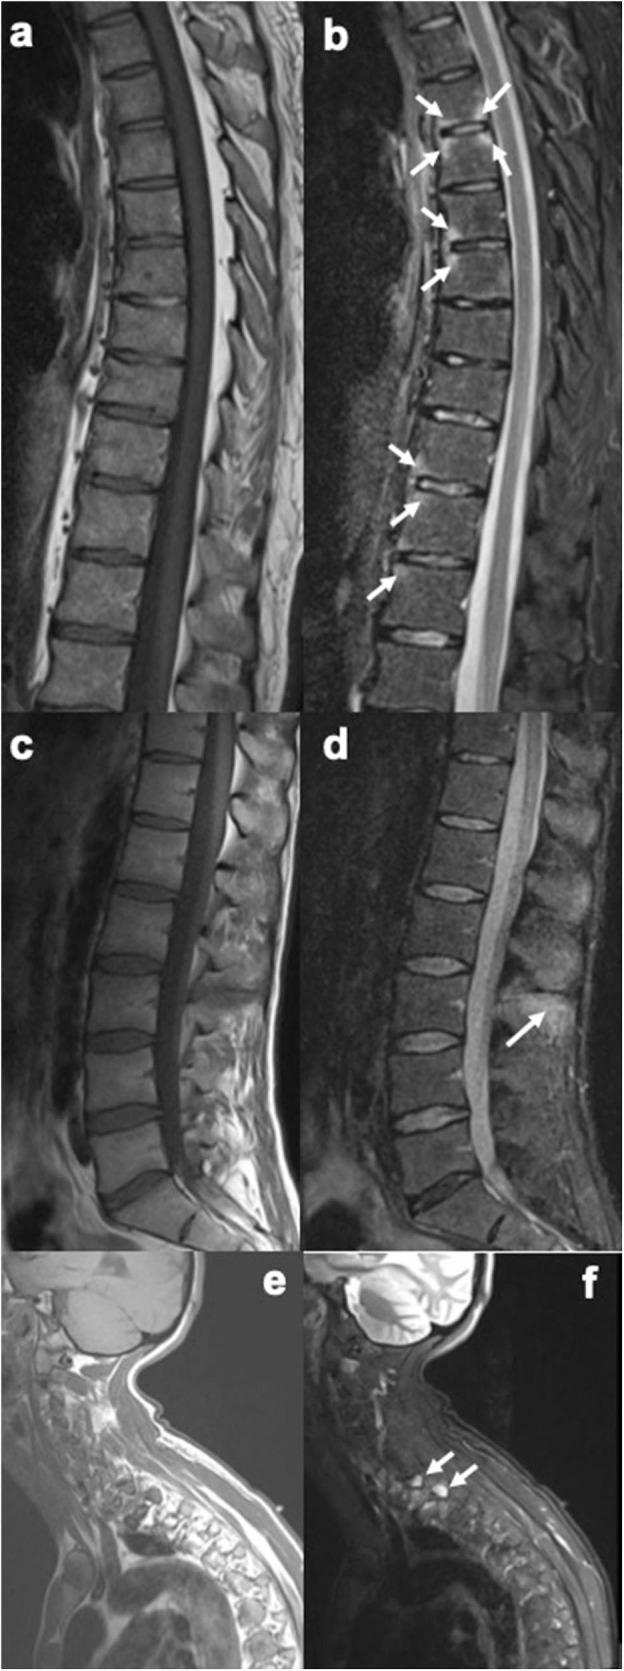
Sagittal MR images of the spine, showing enthesitis at different locations (T1-weighted images on the left, short tau inversion recovery (STIR) images on the right). (a,b) Several anterior and posterior corner inflammatory lesions (arrows) are seen in the thoracic spine, representing enthesitis at the insertion of the anterior and posterior longitudinal ligaments. (c,d) Bone marrow edema is seen at several spinous processes, particularly at the L3 spinous process (arrow), representing enthesitis. (e,f) Bone marrow edema is seen in two upper thoracic transverse processes (arrows), representing enthesitis. Images are from University of Copenhagen, Denmark.
